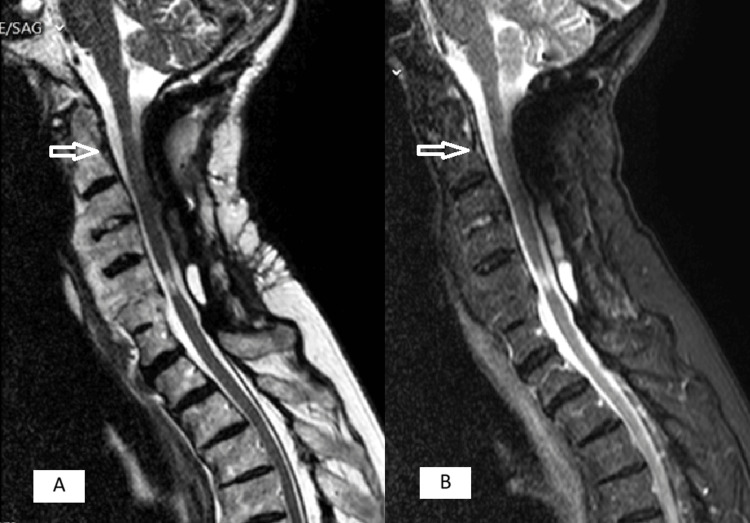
MRI after surgery Arrow shows complete resolution of the periodontoid pannus. (A) T2-weighted image. (B) Short tau inversion recovery (STIR) image
